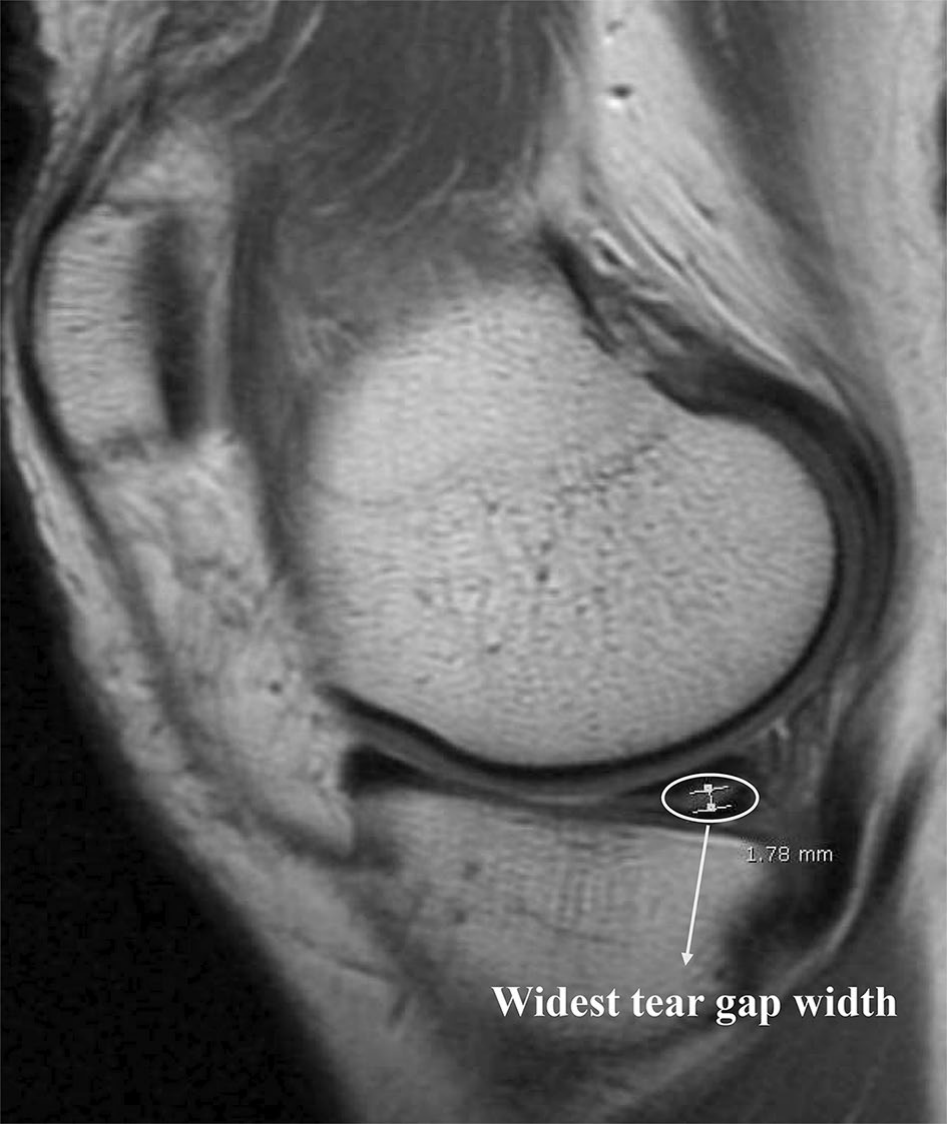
Widest tear gap width measured on the sagittal view of the MRI. Measurements were digitally analyzed via an image analyzing program (Marosis M-view 5.4, Marotech, Seoul, Korea).
